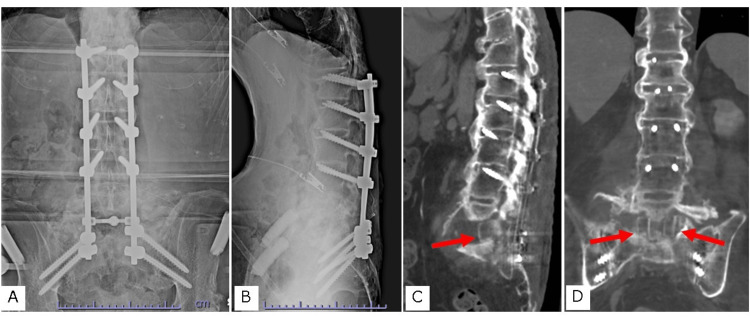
Postoperative X-ray and computed tomography (CT) A. Anteroposterior postoperative X-ray; B. Lateral postoperative X-ray; C. Sagittal plane of lumbosacral CT; D. Coronal plane of CT shows bulk bone grafts
